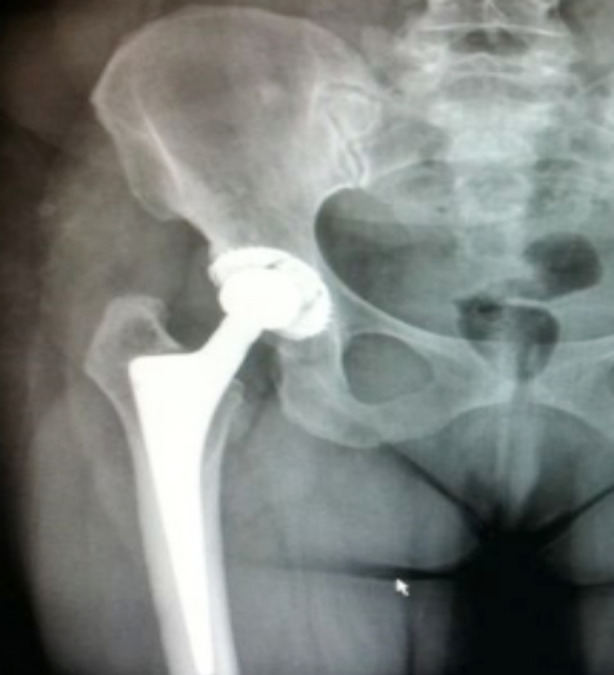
Radiograph of a 64-year-old female patient, showing the 15-year-old implant in THA. The patient had a Harris Hip Score of 96.
